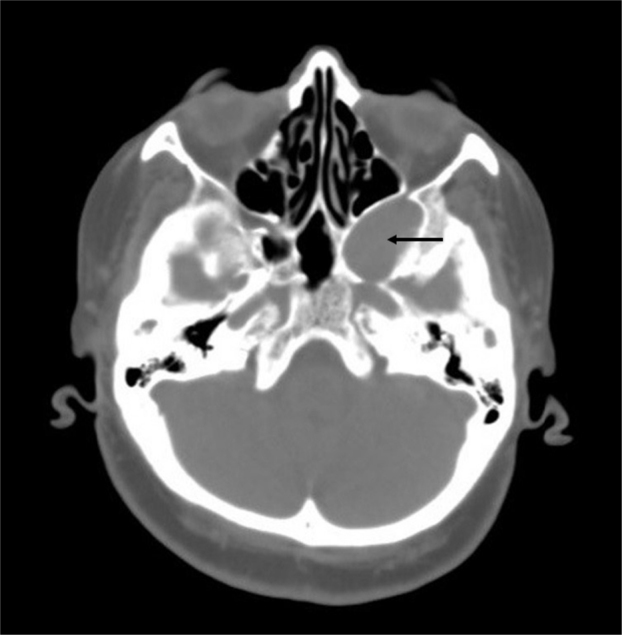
Preoperative axial CT scan. CT scan shows a soft tissue mass, which is located in the pterygopalatine fossa, causing expansion of the left cavernous sinus. Also, it pushes the posterior wall of the maxillary sinus toward the anterior, and the lateral wall of the sphenoid sinus toward the medial (arrow indicates tumor).
